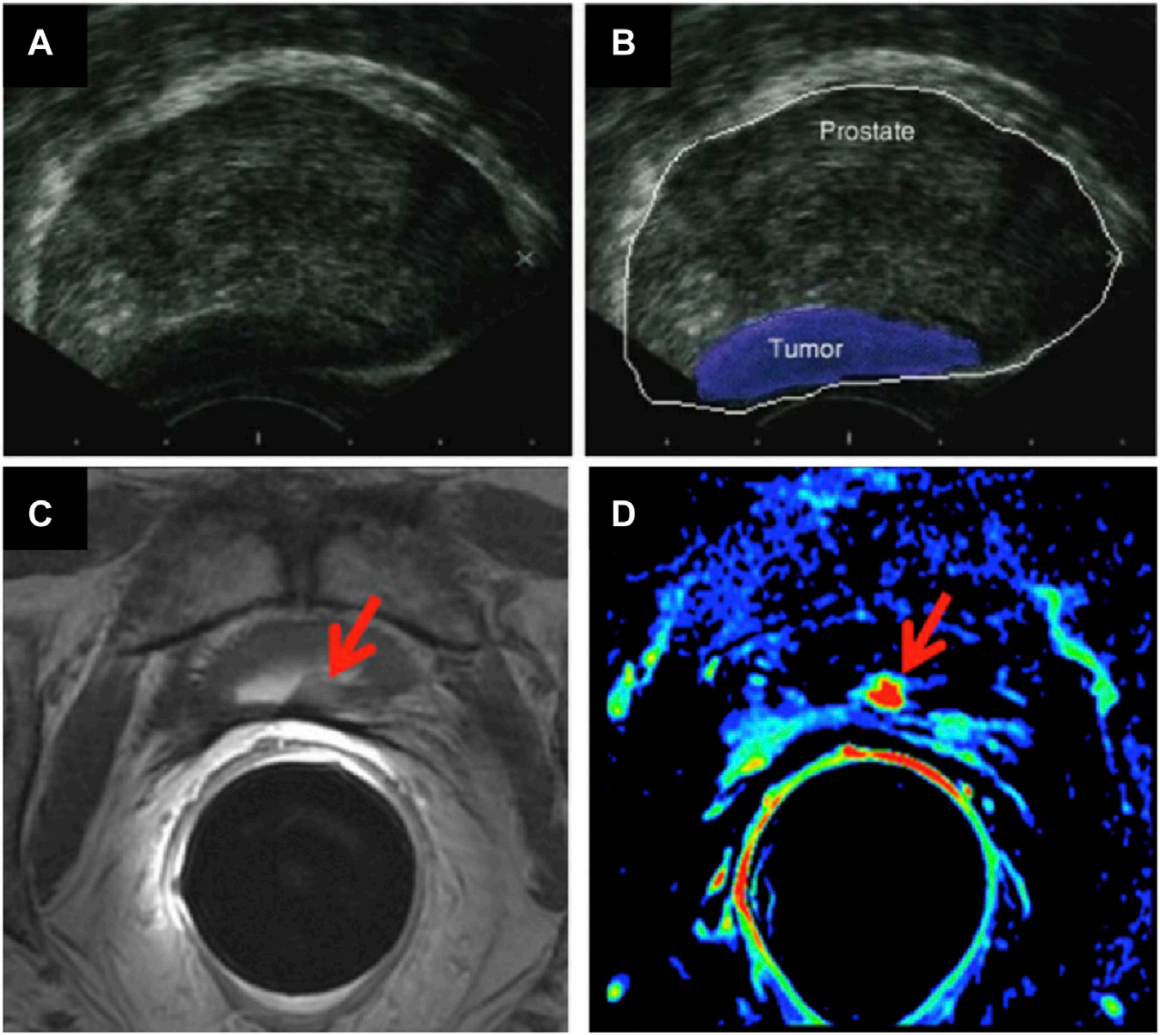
(A,B) TRUS images of a prostate affected by cancer (figure adapted from (Theodorescu and Ehdaie, 2009)); and MRI images of the prostate gland, showing cancerous regions (arrows): (C) Axial T2-weighted fast spin-echo image and (D) axial gradient-echo T1-weighted colour map image (figure adapted from (Maurer et al., 2016)).”
